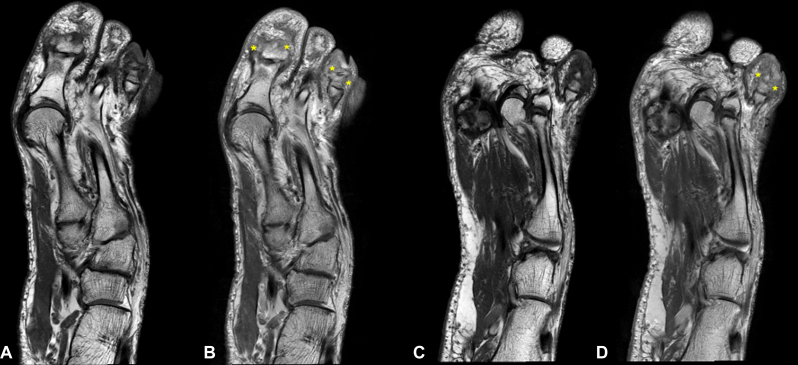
POPP: T1-weighted MRI of the left foot showing soft tissue edema with contrast enhancement postgadolinium (*asterisks*) in the first, third (A and B), and fourth toes (C and D). MRI: pre- (A and C) and post-gadolinium (B and D). *MRI*, Magnetic resonance imaging; *POPP*, psoriatic onycho-pachydermo periostitis.
